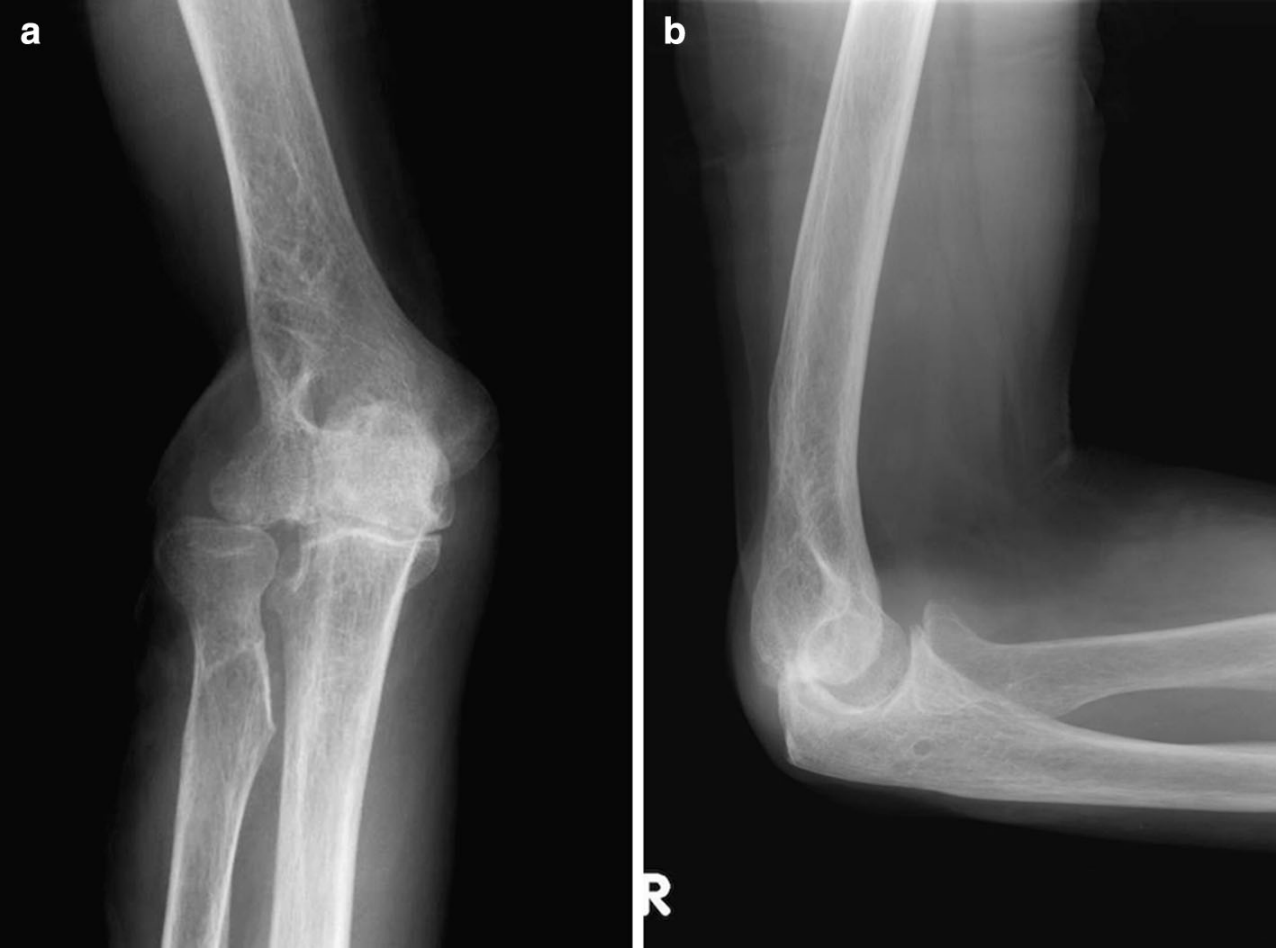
X-rays. a Anteroposterior and b lateral. Degenerative changes in the elbow joint, especially in the radiohumeral joint, were observed 1 year after surgery
